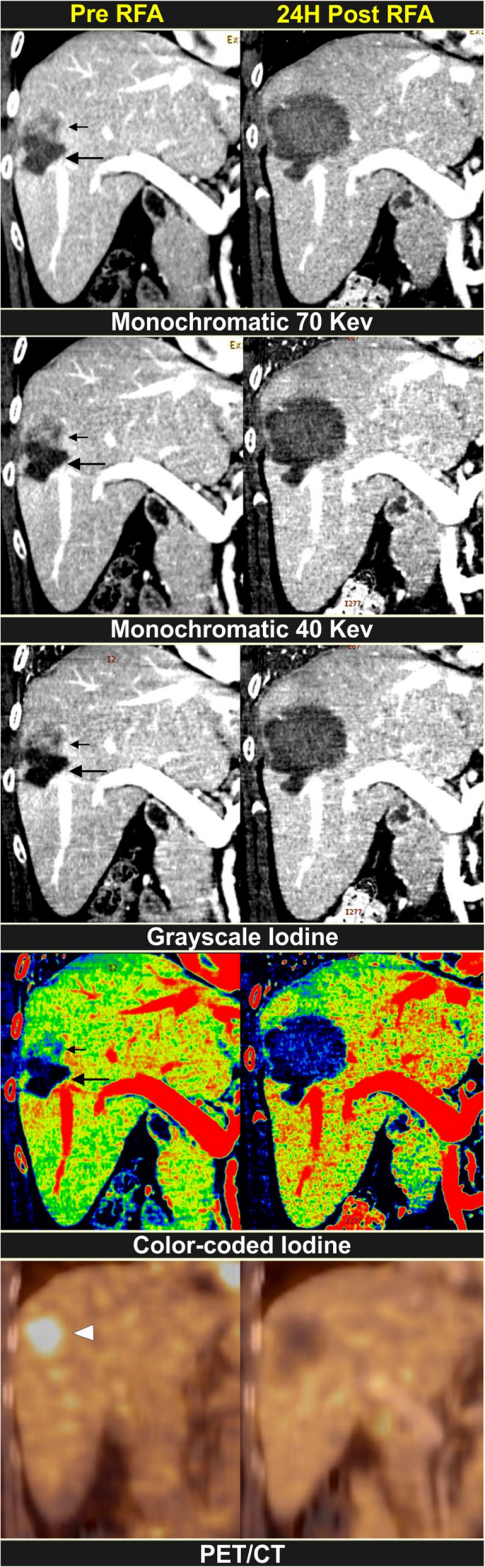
Hypovascular liver metastasis. A 53-year-old female undergoes surgery for rectal carcinoma and then receives RF ablation in a focal liver lesion. Eight months later, a local tumour progression (first column) at the cranial side of the ablation zone (*large arrow*) can be seen as an intermediate density (*small arrow*). In this straightforward case, the tumoral tissue is equally well depicted on the 40- and 70-keV images as on the greyscale iodine images. Noise is more prominent on the 40-keV image however. Iodine maps are encoded using a rainbow template with a colour coding from high to low iodine concentration, being from red (through yellow) to blue respectively. The intermediate colour represents a tumour (*small arrow*). On PET/CT a vivid FDG uptake is seen (*arrowhead*). Twenty-four hours after a second RF ablation (second column), a sharp delineated ablation zone is found. On all reconstructions, the avascular nature of the ablation zone is well demonstrated. The contrast with the surrounding liver parenchyma appears highest on the colour-coded iodine map. No FDG uptake is seen on the PET/CT image. The PET/CT 3 months later (not shown) does not show signs of tumoral activity
